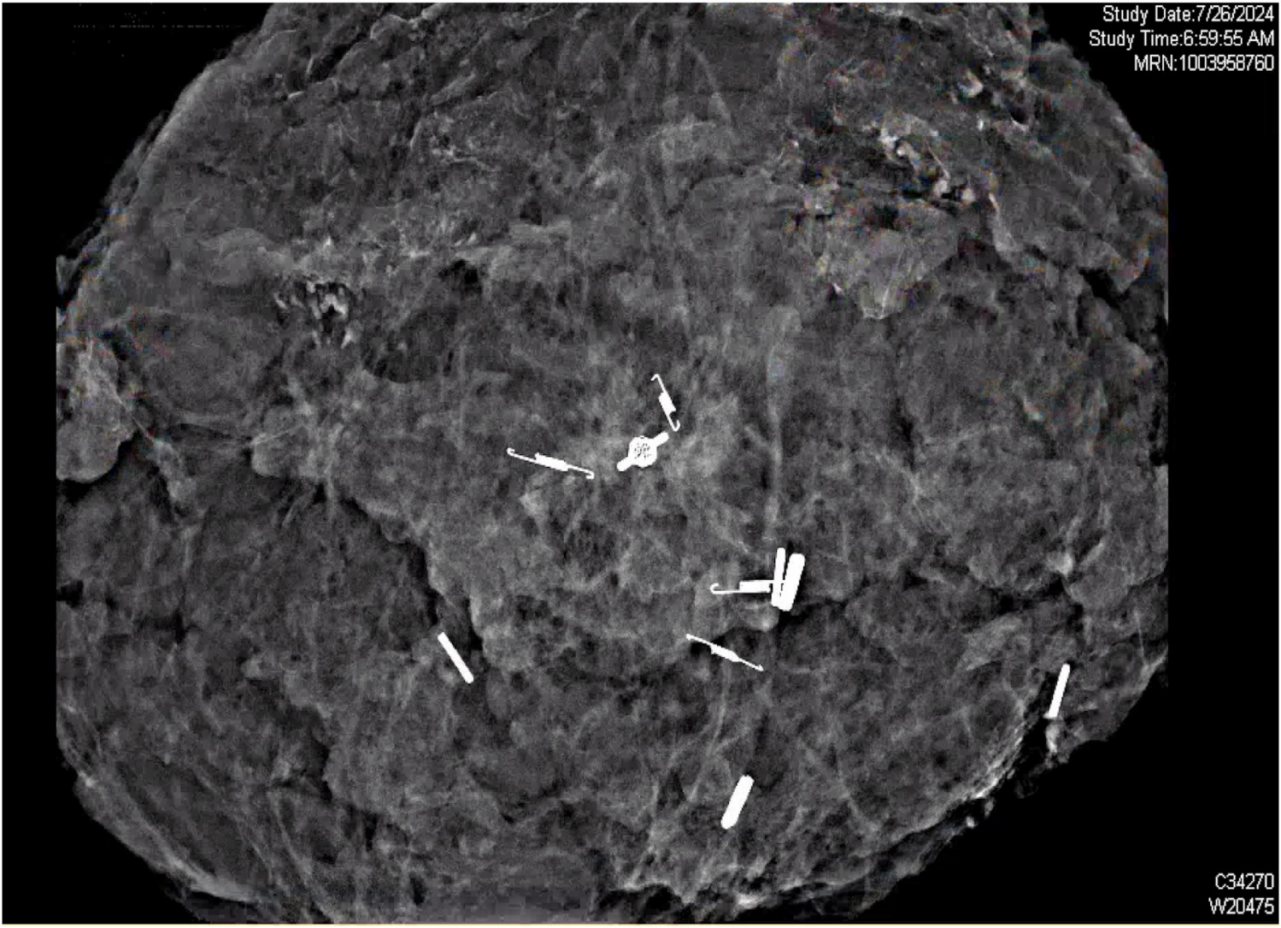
Post-resection ultrasound scan of the mass, identifying the four unique SCOUT reflectors surrounding the punctate calcifications in excised tissue.
